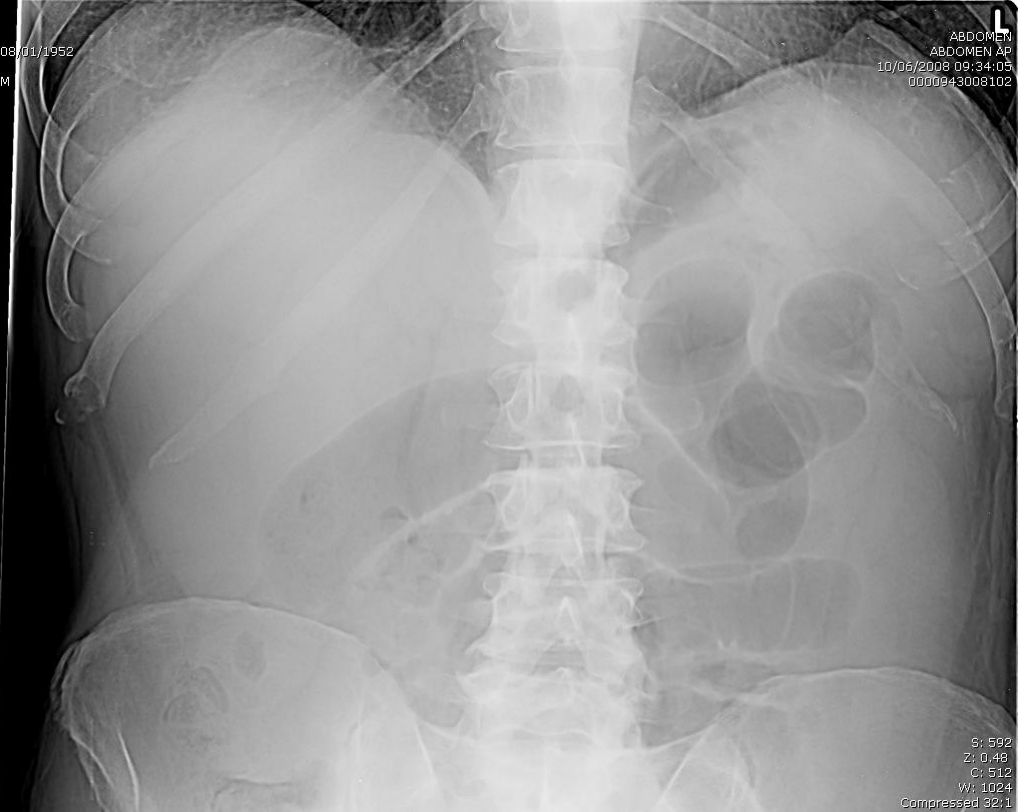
Plain abdominal x-ray. Distended small bowel loops consistent with small bowel obstruction.
